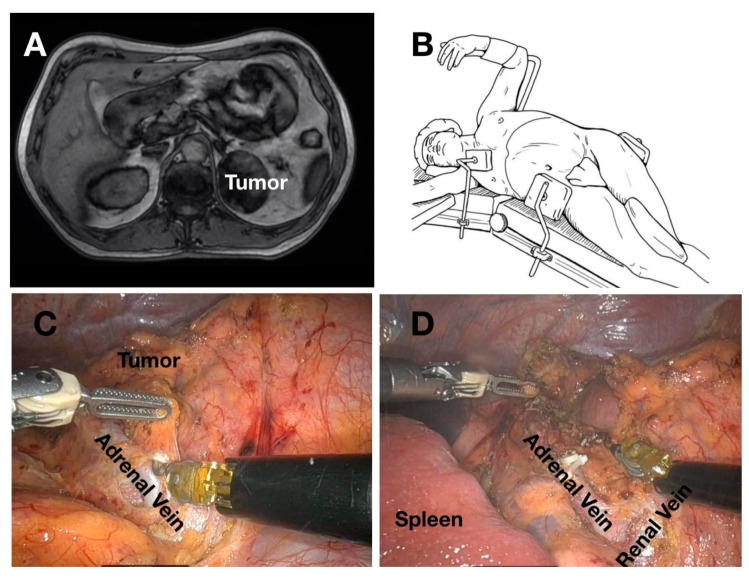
Left adrenal metastasis evidentiated on MRI (A); patient position on the table for left robotic adrenalectomy (B); robotic dissection from surrounding structures (C), and final intraoperative view (D).
